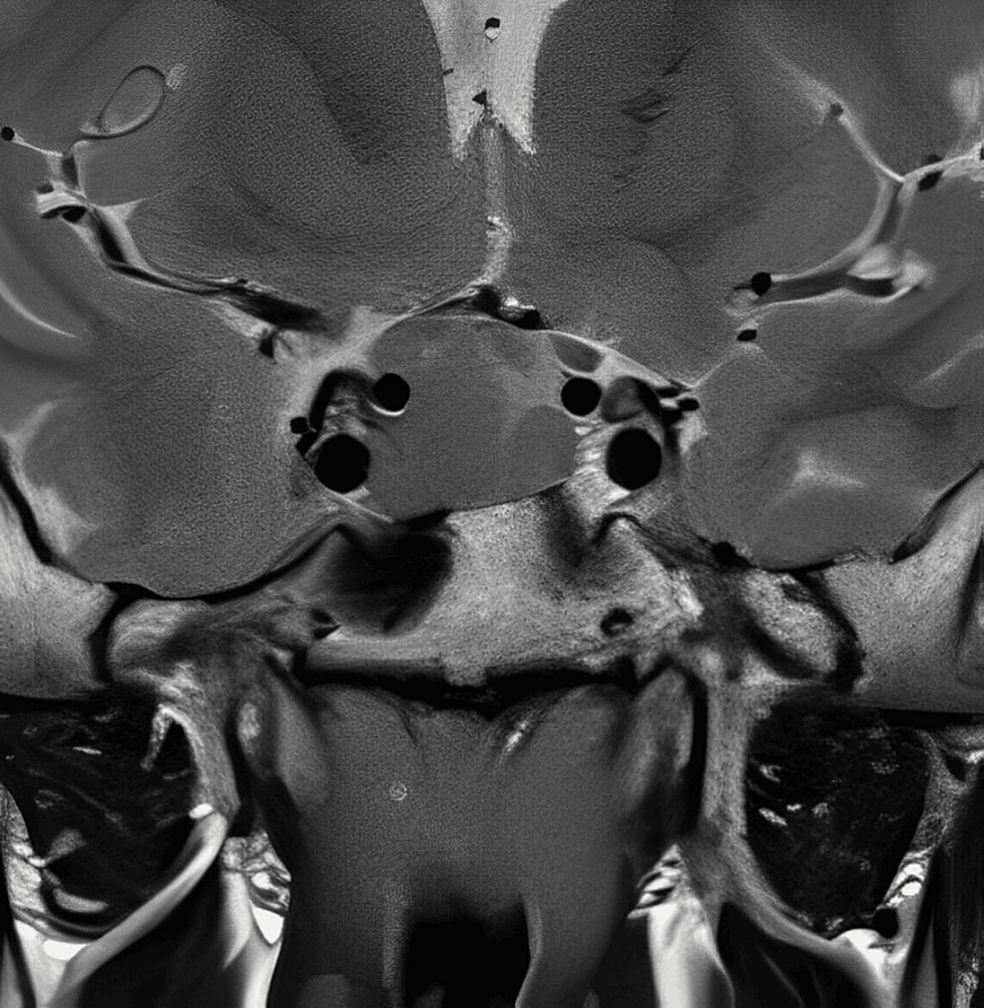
Coronal T2-weighted magnetic resonance image of the brain in an 18-year-old patient showing pituitary macroadenoma.
